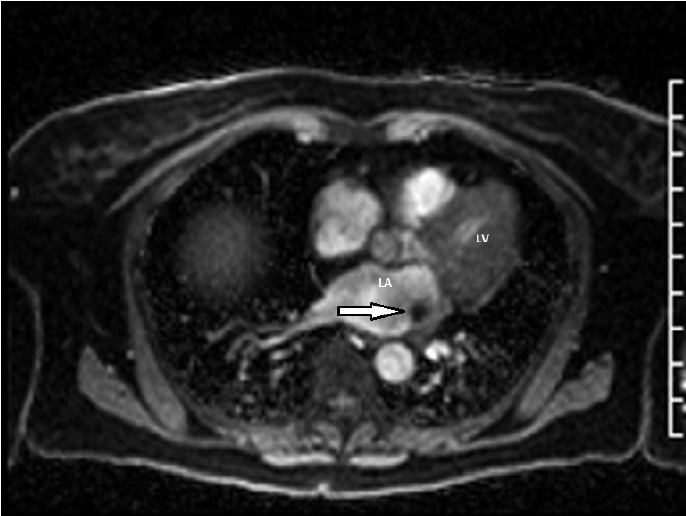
The procedure was performed via median sternotomy with the aid of cardiopulmonary bypass. Central aortic arterial cannulation with bicaval venous cannulation was performed. The heart was arrested with aid of antegrade cardioplegia. For surgical exposure, Sondergaard’s groove was dissected and a left atriotomy performed. A 1 cm mobile, gelatinous mass on the left atrial wall was found (Figure 3). This arose between the left atrial appendage and the left superior pulmonary vein. The lesion was resected en bloc, along with the stalk and a margin of the left atrial wall. The defect was closed using a 4-0 monofilament suture. The immediate post-operative course was uneventful and the patient was discharged home on post-operative day 4. On outpatient follow up she has been doing well and has remained asymptomatic. A repeat echocardiogram is scheduled to be performed at one year follow up.
